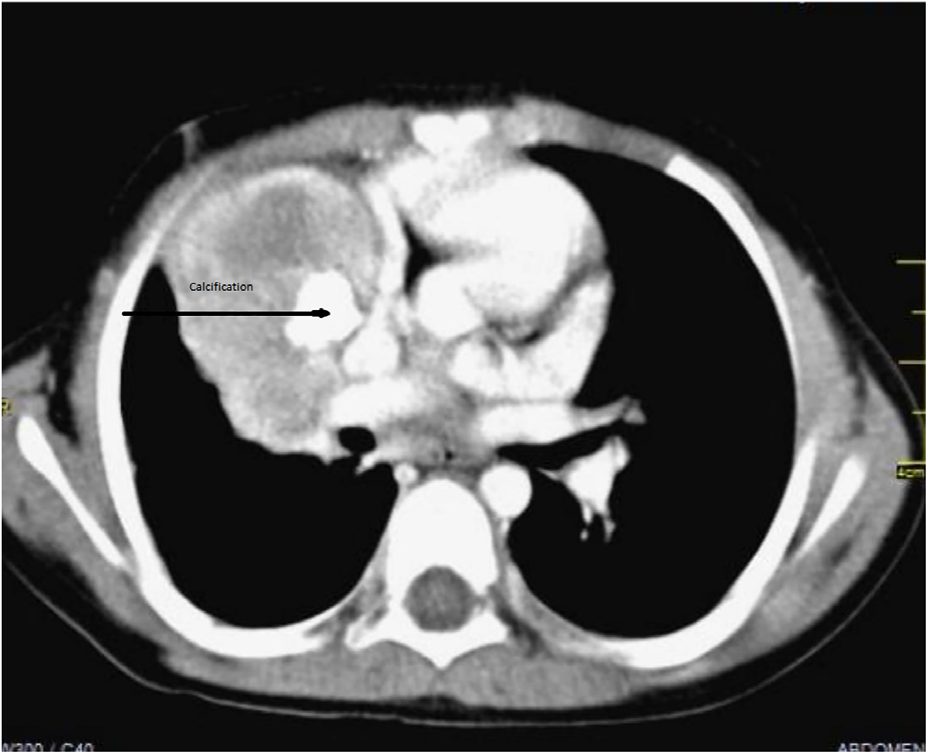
Computed tomography of the myofibroblastic tumour of the lung
